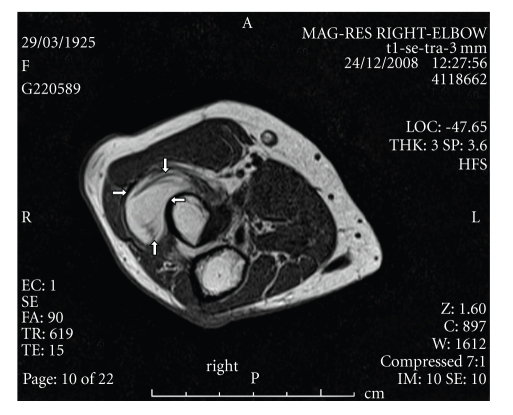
T1-weighted axial MR images reveal a high-intensity lesion around the radius, white arrows.
